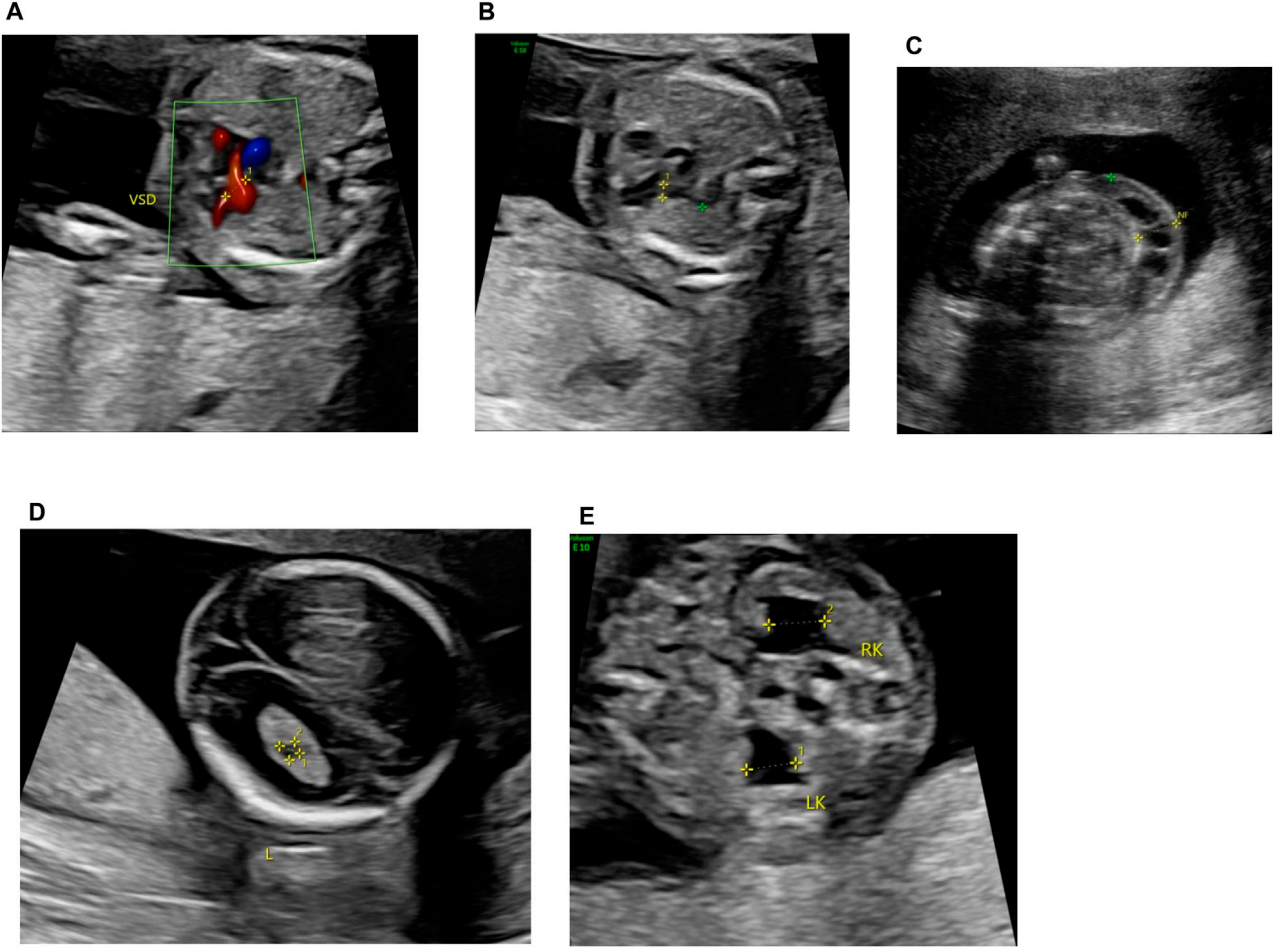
Fetal ultrasonography (at 16 weeks of gestation). (A) VSD, (B) pulmonary stenosis, (C) CCH, (D) choroid plexus cyst, and (E) bilateral hydronephrosis.
